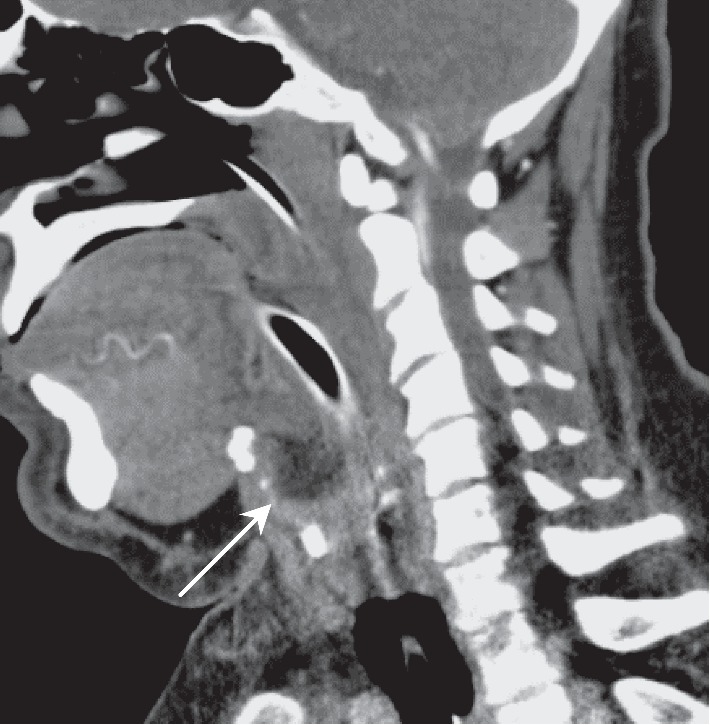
Sagittal view of CT neck with contrast demonstrating an extensive parapharyngeal soft tissue swelling and oedema, with an arrow pointing to the epiglottic abscess formation.
